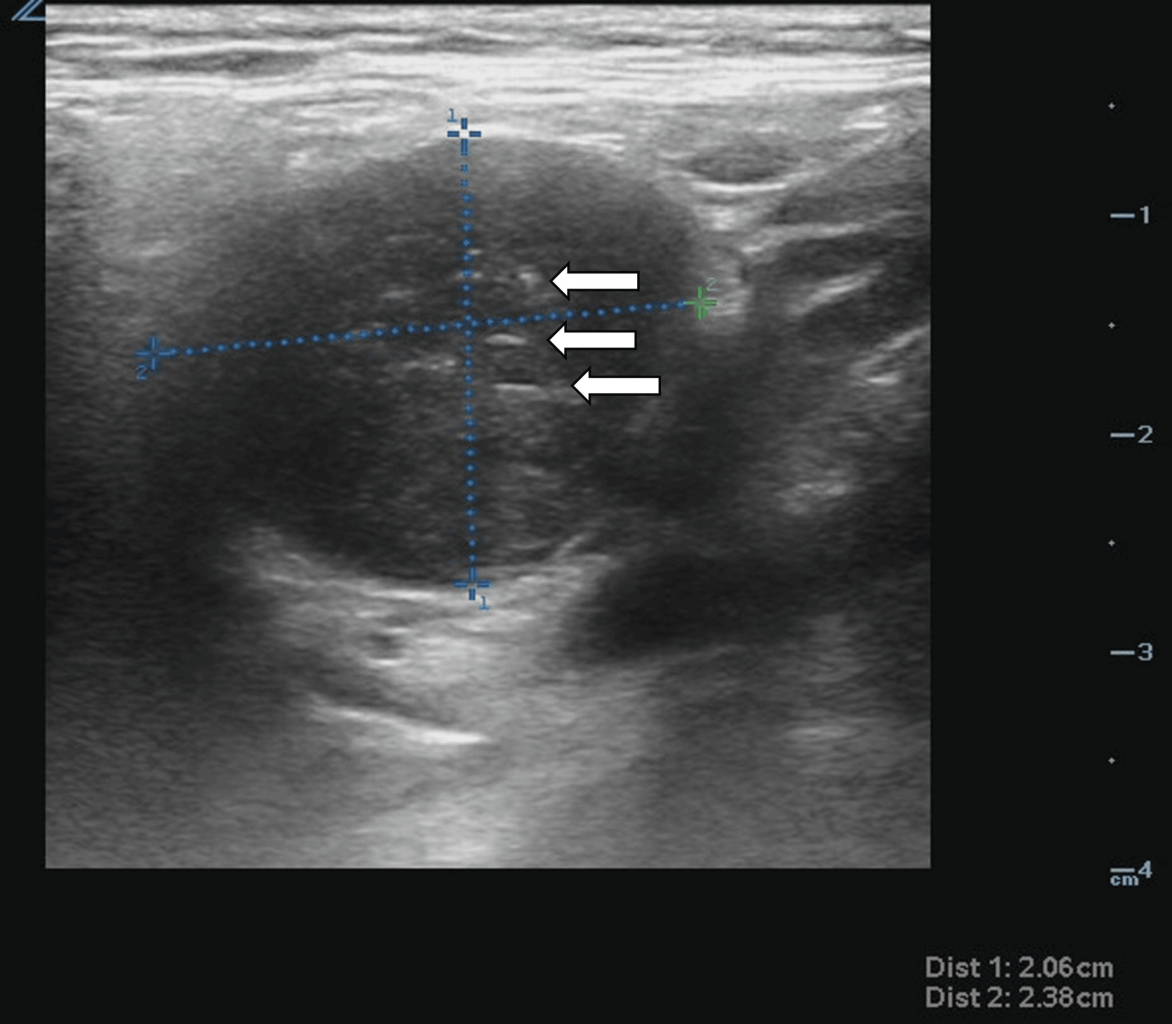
Enlarged cervical lymph node The ultrasound image shows an enlarged lymph node with a heterogenous appearance, obliteration of normal structure, increased blood flow on the Doppler, and a size of 2.1 x 2.4 cm. The mixed hyperechoic (white arrows) and isoechoic nature of the lymph node, added to the above other features like >2 cm size and abnormal structure, would mark this lymph node as "abnormal" in a typical PoCUS evaluation. An "abnormal" lymph node in point-of-care testing helps increase the clinician's suspicion of malignancy and guide which lymph nodes should be biopsied.
